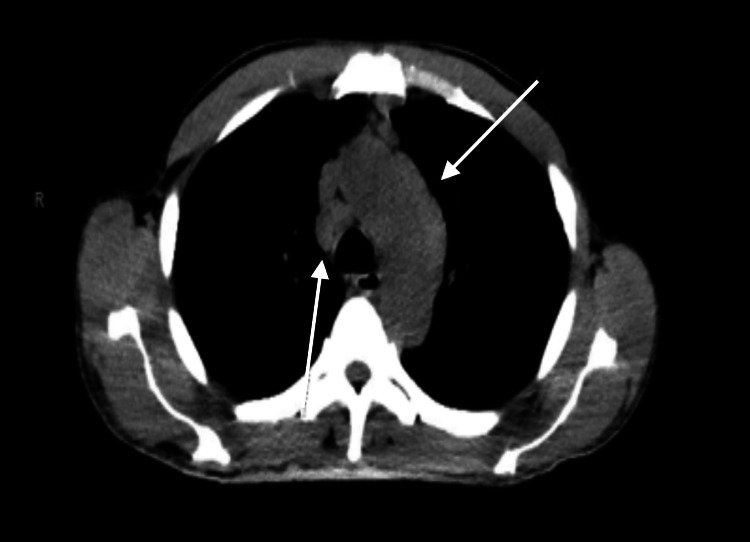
Computed tomography of the chest indicating bilateral hilar lymphadenopathy
